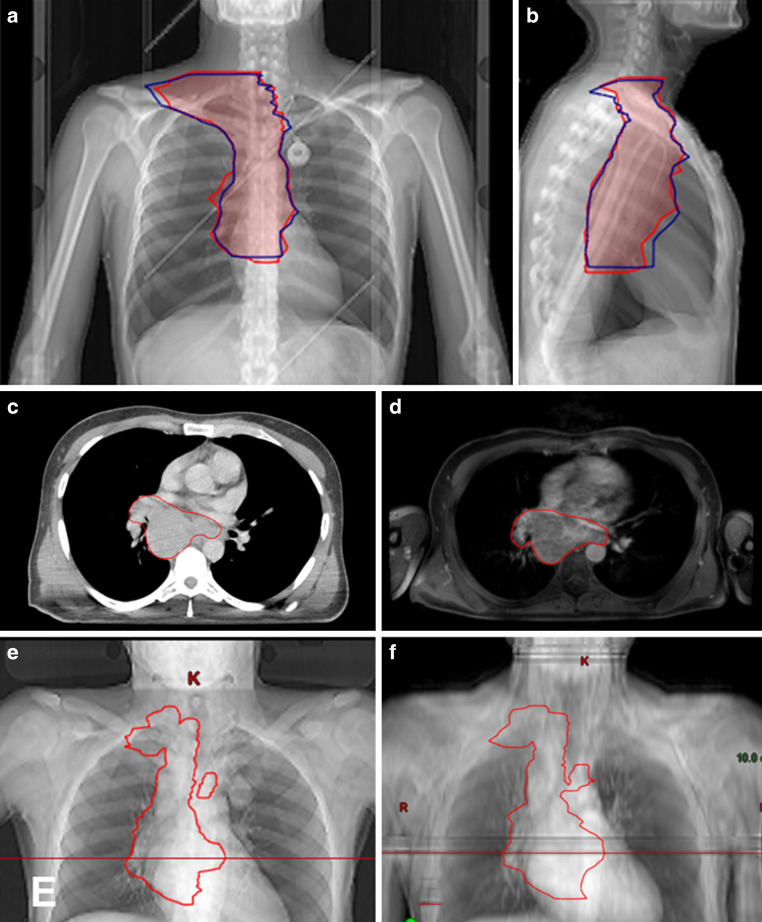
GTV and CTV comparison (patient 4). a, b Red area: CTV based on PET/CT. Blue outline: CTV based on PET/MRI. a frontal view, b lateral view. c, d: Red area: GTV based on PET/CT (c) and PET/MRI (d). e, f Corresponding topograms with marking of the section plane
